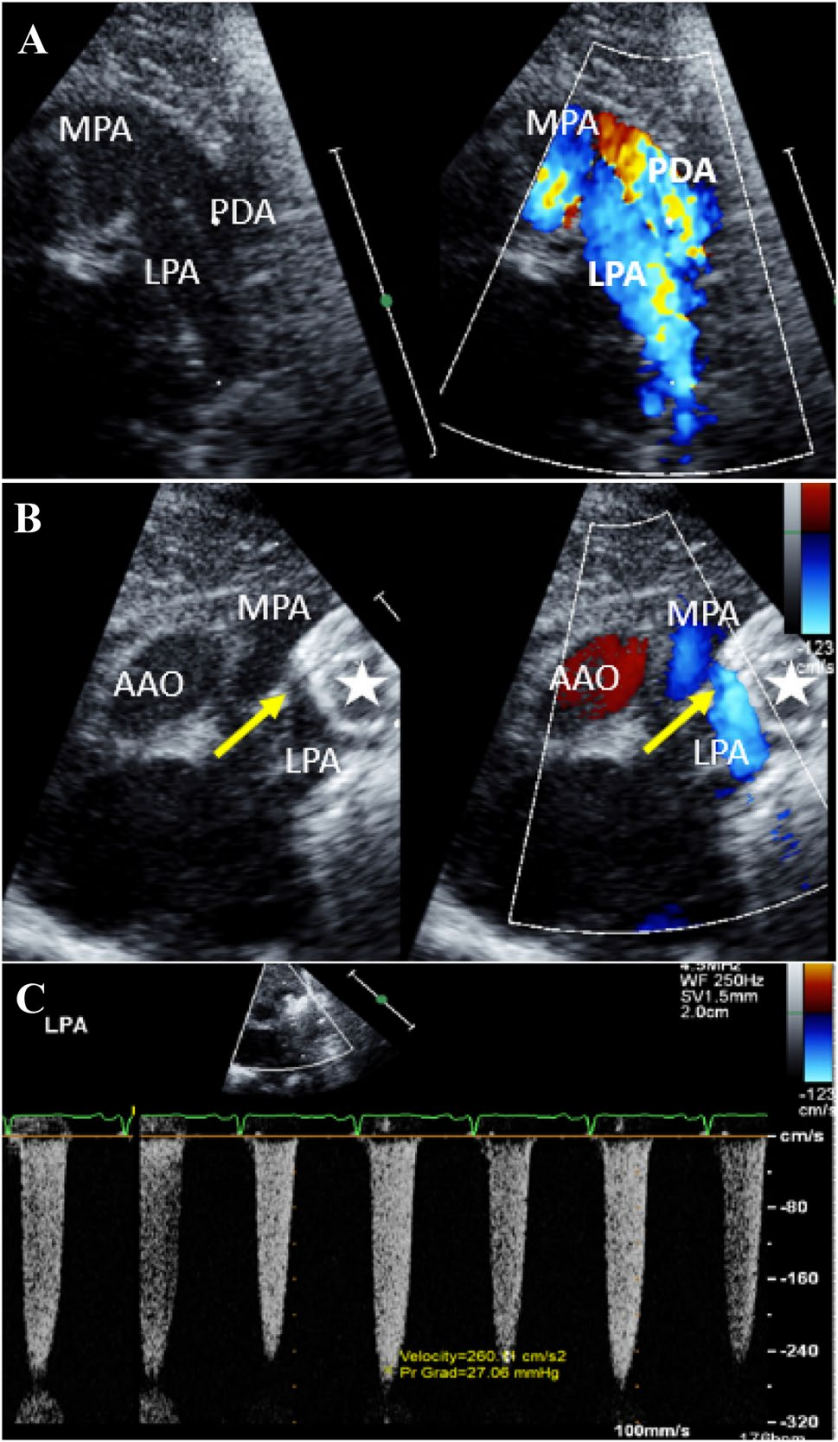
Patient who developed LPA obstruction. Echocardiographic images of the patient who developed device-related LPA stenosis. a 2D and color Doppler images side-by-side at baseline, before device PDA closure. A large tubular PDA is visualized with a parallel orientation to the LPA, and shunting seen across the PDA from the DAO into the distal main pulmonary artery. b, c Intraprocedural images immediately post device closure showing the proximal portion of the AVP II device (white star) compressing the proximal LPA (yellow arrow), resulting in an elevated peak LPA velocity of 2.6 m/s, despite not demonstrating a classic obstructive flow pattern of continuous diastolic flow
